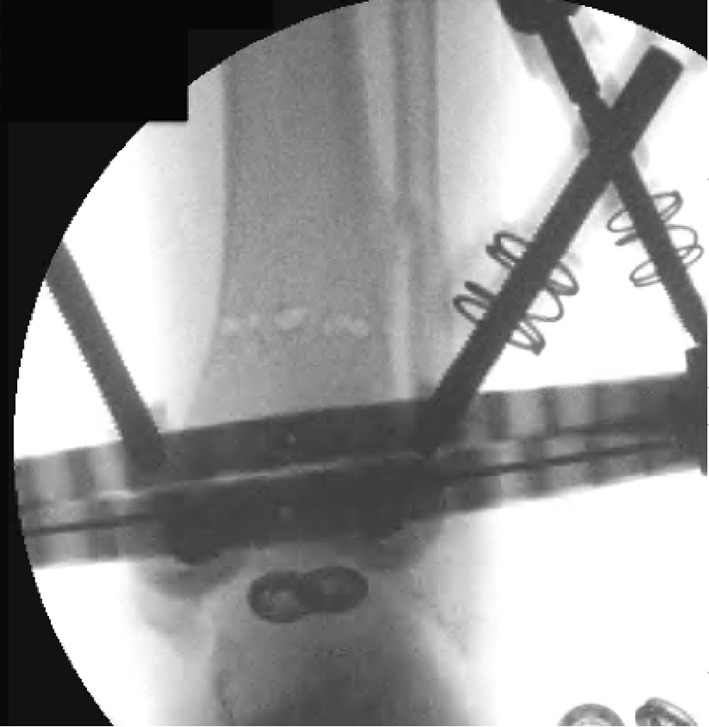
Clinical radiograph demonstrating the appearance of the distal tibia following the result of the multiple drill hole osteotomy being performed
